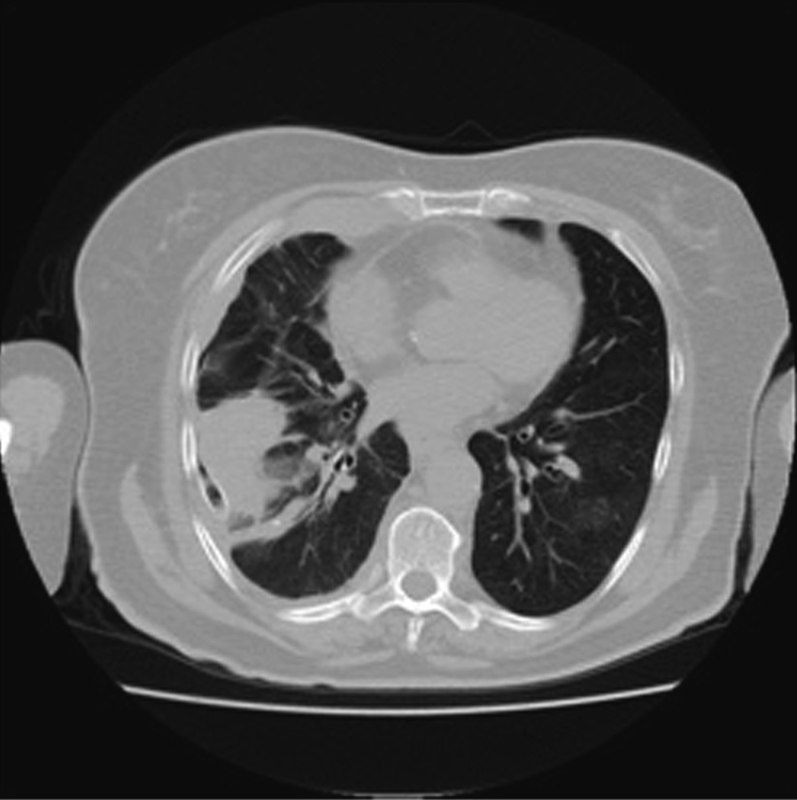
Computed tomography (CT) showing pleural thickening in the right hemithorax and pleural effusion.
